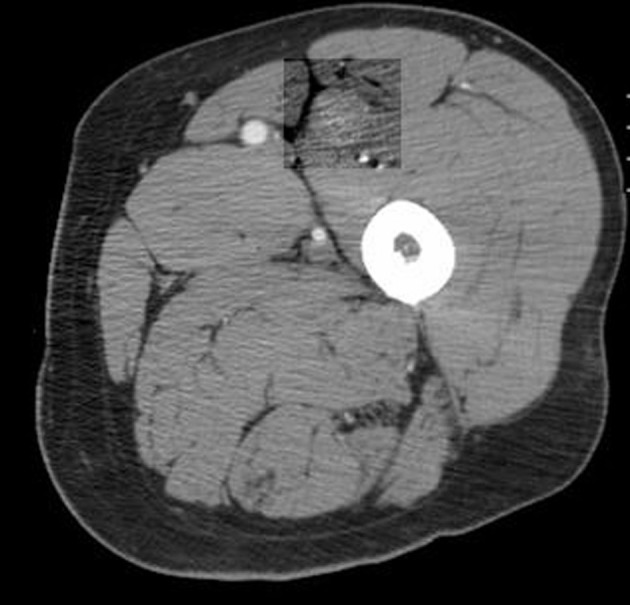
CT scan of the left thigh.
